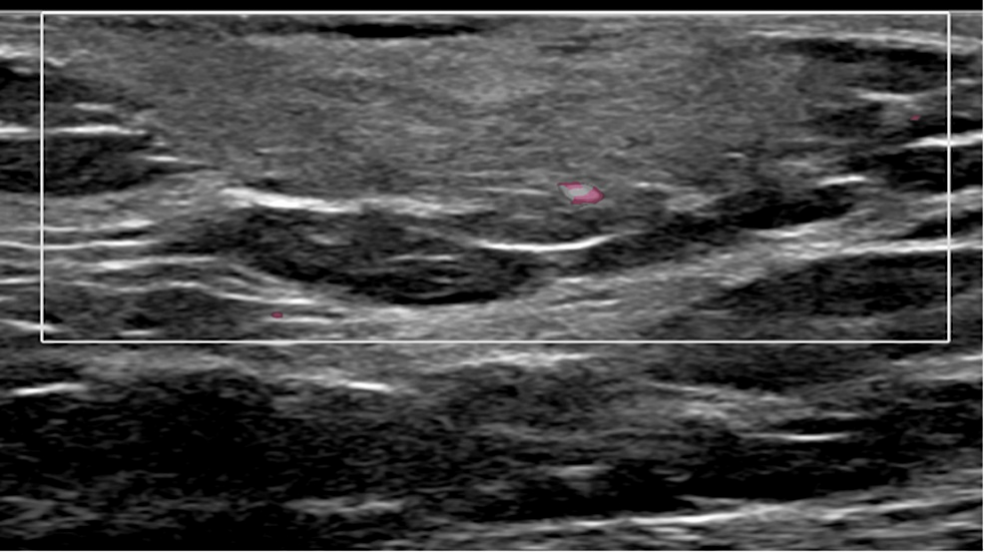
Color Doppler ultrasound image of the left breast palpable mass demonstrates mild internal vascularity.
